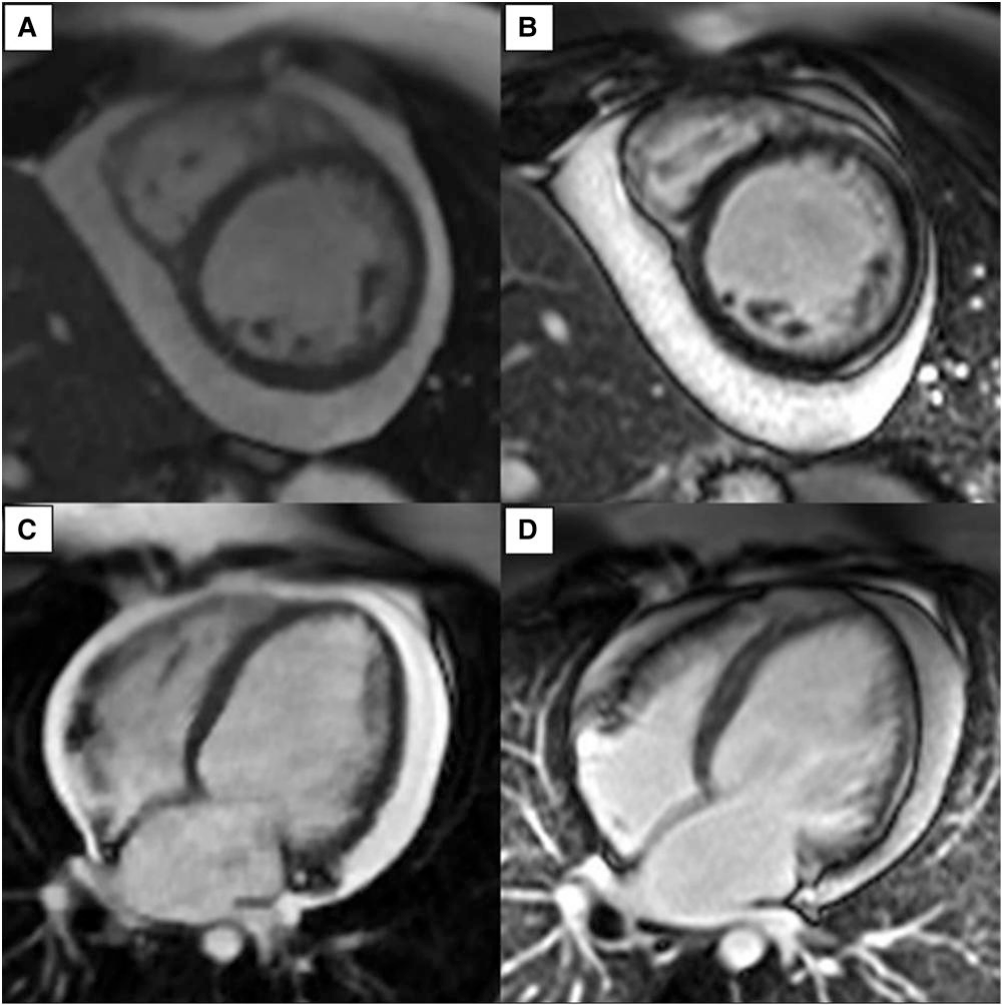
Cardiac magnetic resonance images. (*A*) Cine short-axis image at the mid left ventricle. (*B*) Corresponding late gadolinium enhancement short-axis image shows subepicardial hyperenhancement at the lateral segment. (*C*) Cine horizontal long-axis image. (*D*) Corresponding late gadolinium enhancement horizontal long-axis image shows subepicardial hyperenhancement at the lateral segment and mid-wall hyperenhancement at the septal segment. All images show moderate circumferential pericardial effusion.
